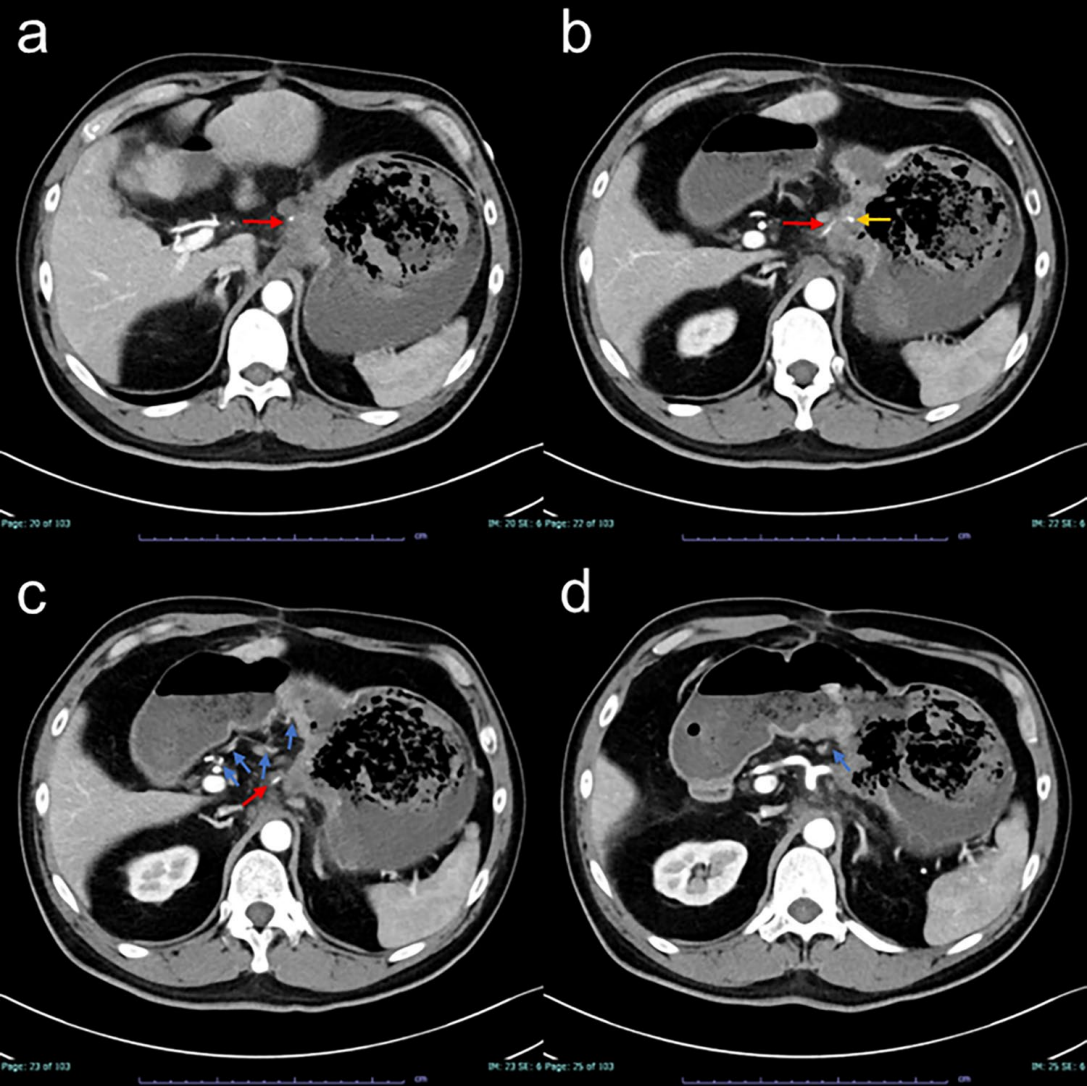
The arterial phase contrast-enhanced computed tomography images on the day of bleeding from gastric cancer (a–d, from cranial to caudal side). The arrows indicate the common trunk of the left gastric and the left hepatic arteries in red, the right gastric artery in blue, and the pseudoaneurysm in yellow. The pseudoaneurysm originated from the left gastric artery. The gastric tumor located at the lesser curvature of the stomach was blood supplied by both the left and the right gastric arteries
